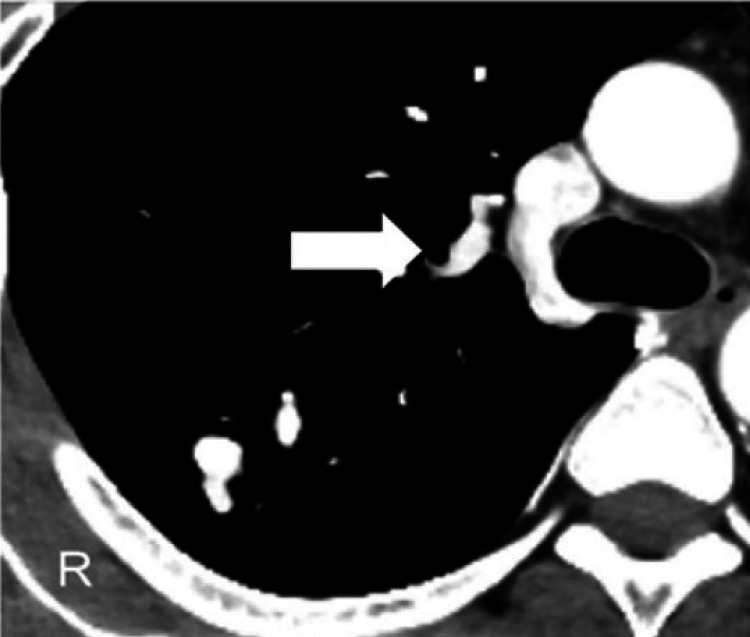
Contrast-enhanced computed tomography scan of the chest on day 64. A follow-up computed tomography confirms the disappearance of the thrombus (white arrow).
